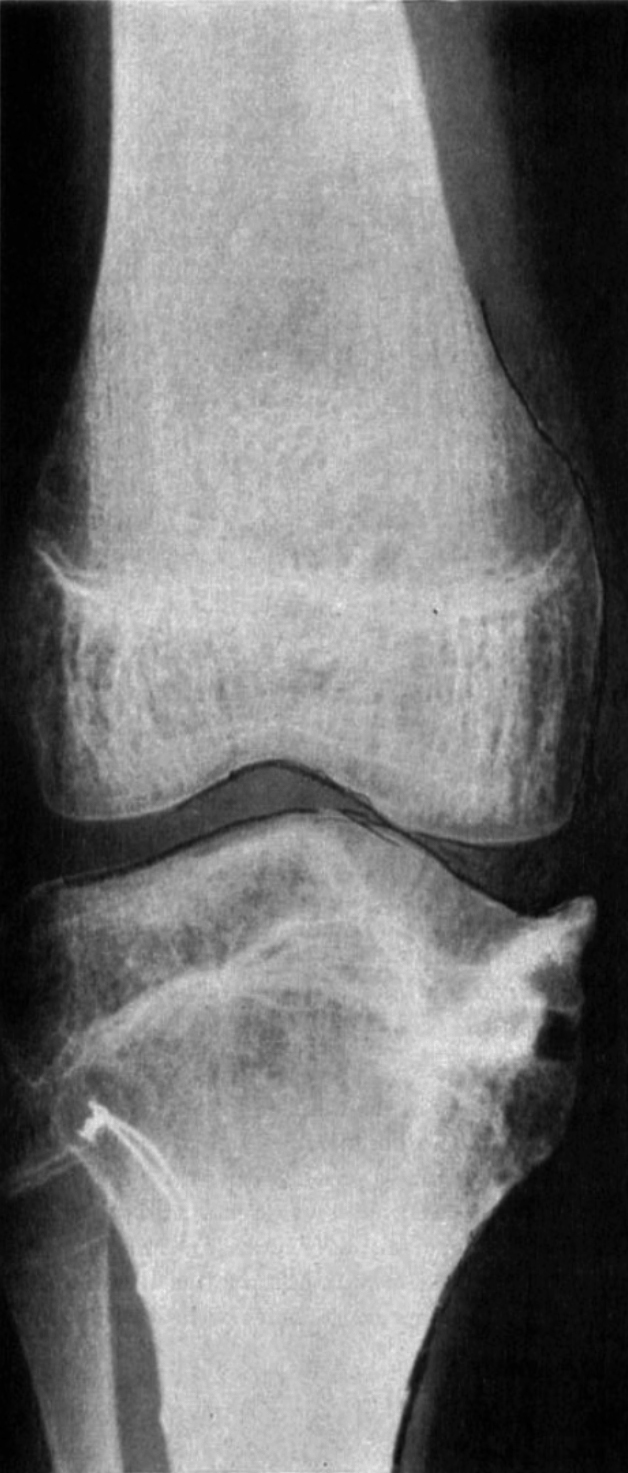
Radiograph 1 year after operative elevation of the medial tibial joint surface, showing consolidation and good correction of the varus deformity.
